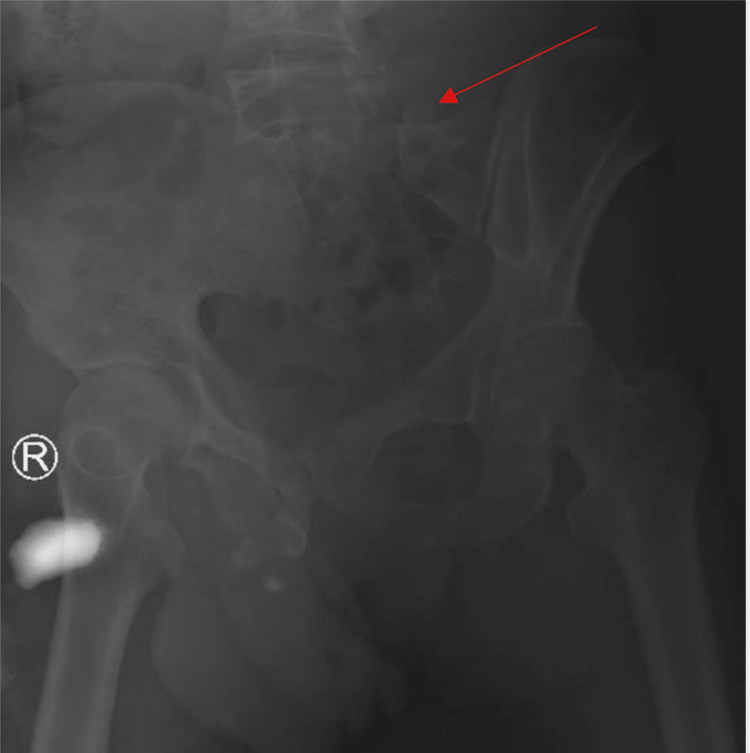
Anteroposterior (AP) X-ray of the pelvis. The arrow shows transforaminal fracture of the left sacrum (Denis type II) with proximal migration and displacement.
